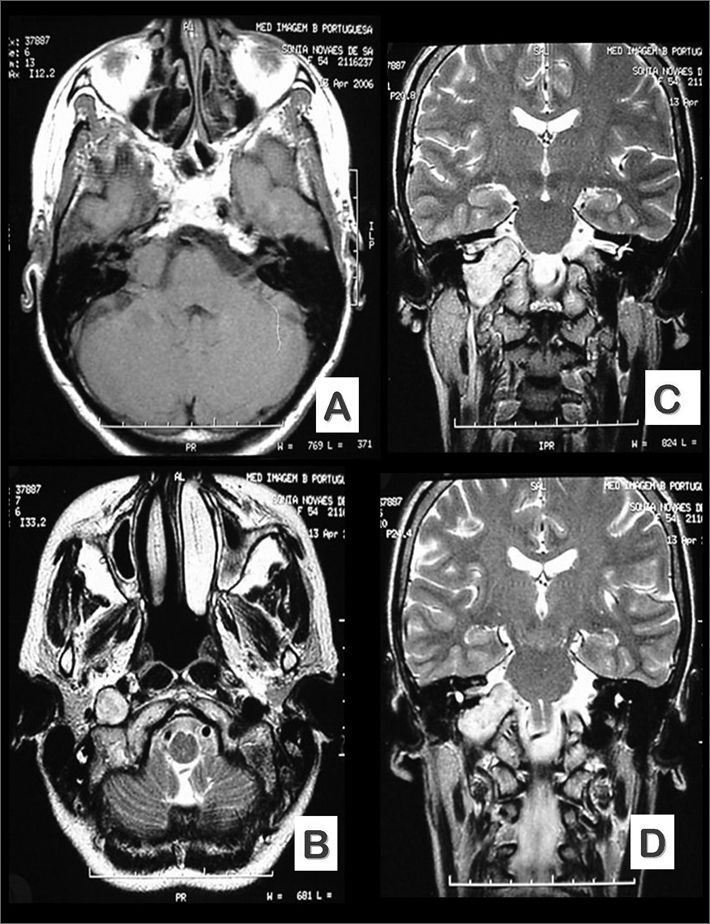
Preoperative MRI images (A and B - axial views, and C and D - coronal views) showing lesion location and size.
